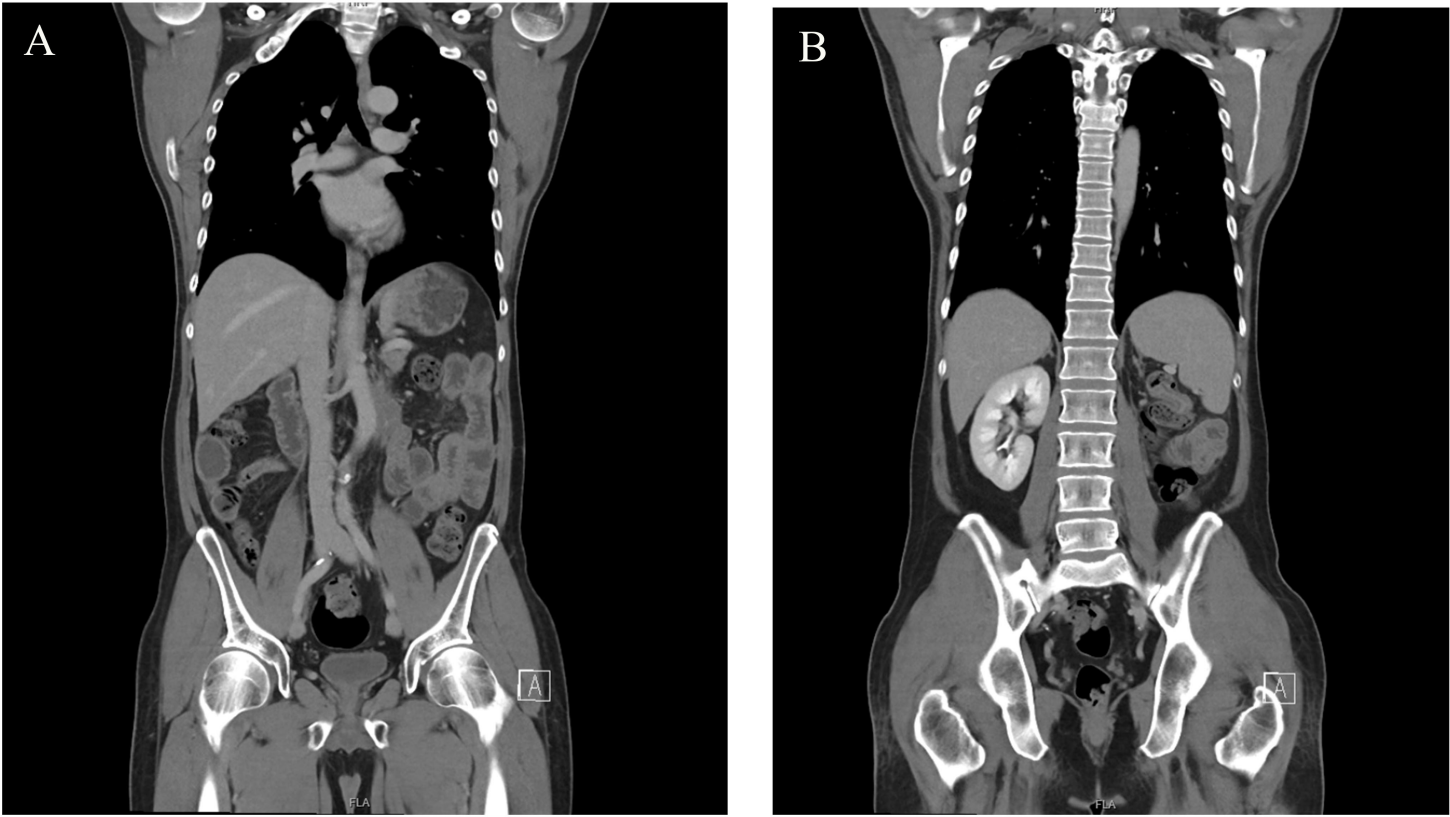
(A, B) Four months post-nephrectomy, contrast-enhanced abdominal CT with three-dimensional reconstruction demonstrated no abnormal lymphadenopathy or masses in the abdominal cavity.
